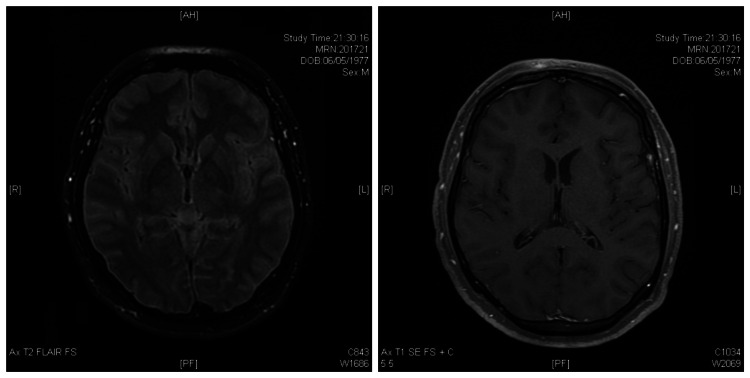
Brain magnetic resonance imaging (FLAIR and post-contrast T1) showing no parenchymal or meningeal abnormalities. Brain magnetic resonance imaging performed on day 0 (Figure 1). (A) Axial T2-weighted fluid-attenuated inversion recovery (FLAIR) with fat suppression demonstrating normal brain parenchyma with no focal signal abnormalities. The absence of cerebrospinal fluid signal suppression is attributed to a technical artifact related to high inspired oxygen fraction during mechanical ventilation and does not reflect true inflammatory or proteinaceous changes. (B) Axial T1-weighted spin-echo with fat suppression following gadolinium administration (T1 SE FS+C) showing no pathological leptomeningeal or parenchymal enhancement. Diffusion-weighted imaging (not shown) demonstrated no areas of restricted diffusion. These findings are consistent with the "E-MRI" phenotype described in SARS-CoV-2 encephalitis, in which a confirmed CNS infection may occur despite normal neuroimaging findings.
